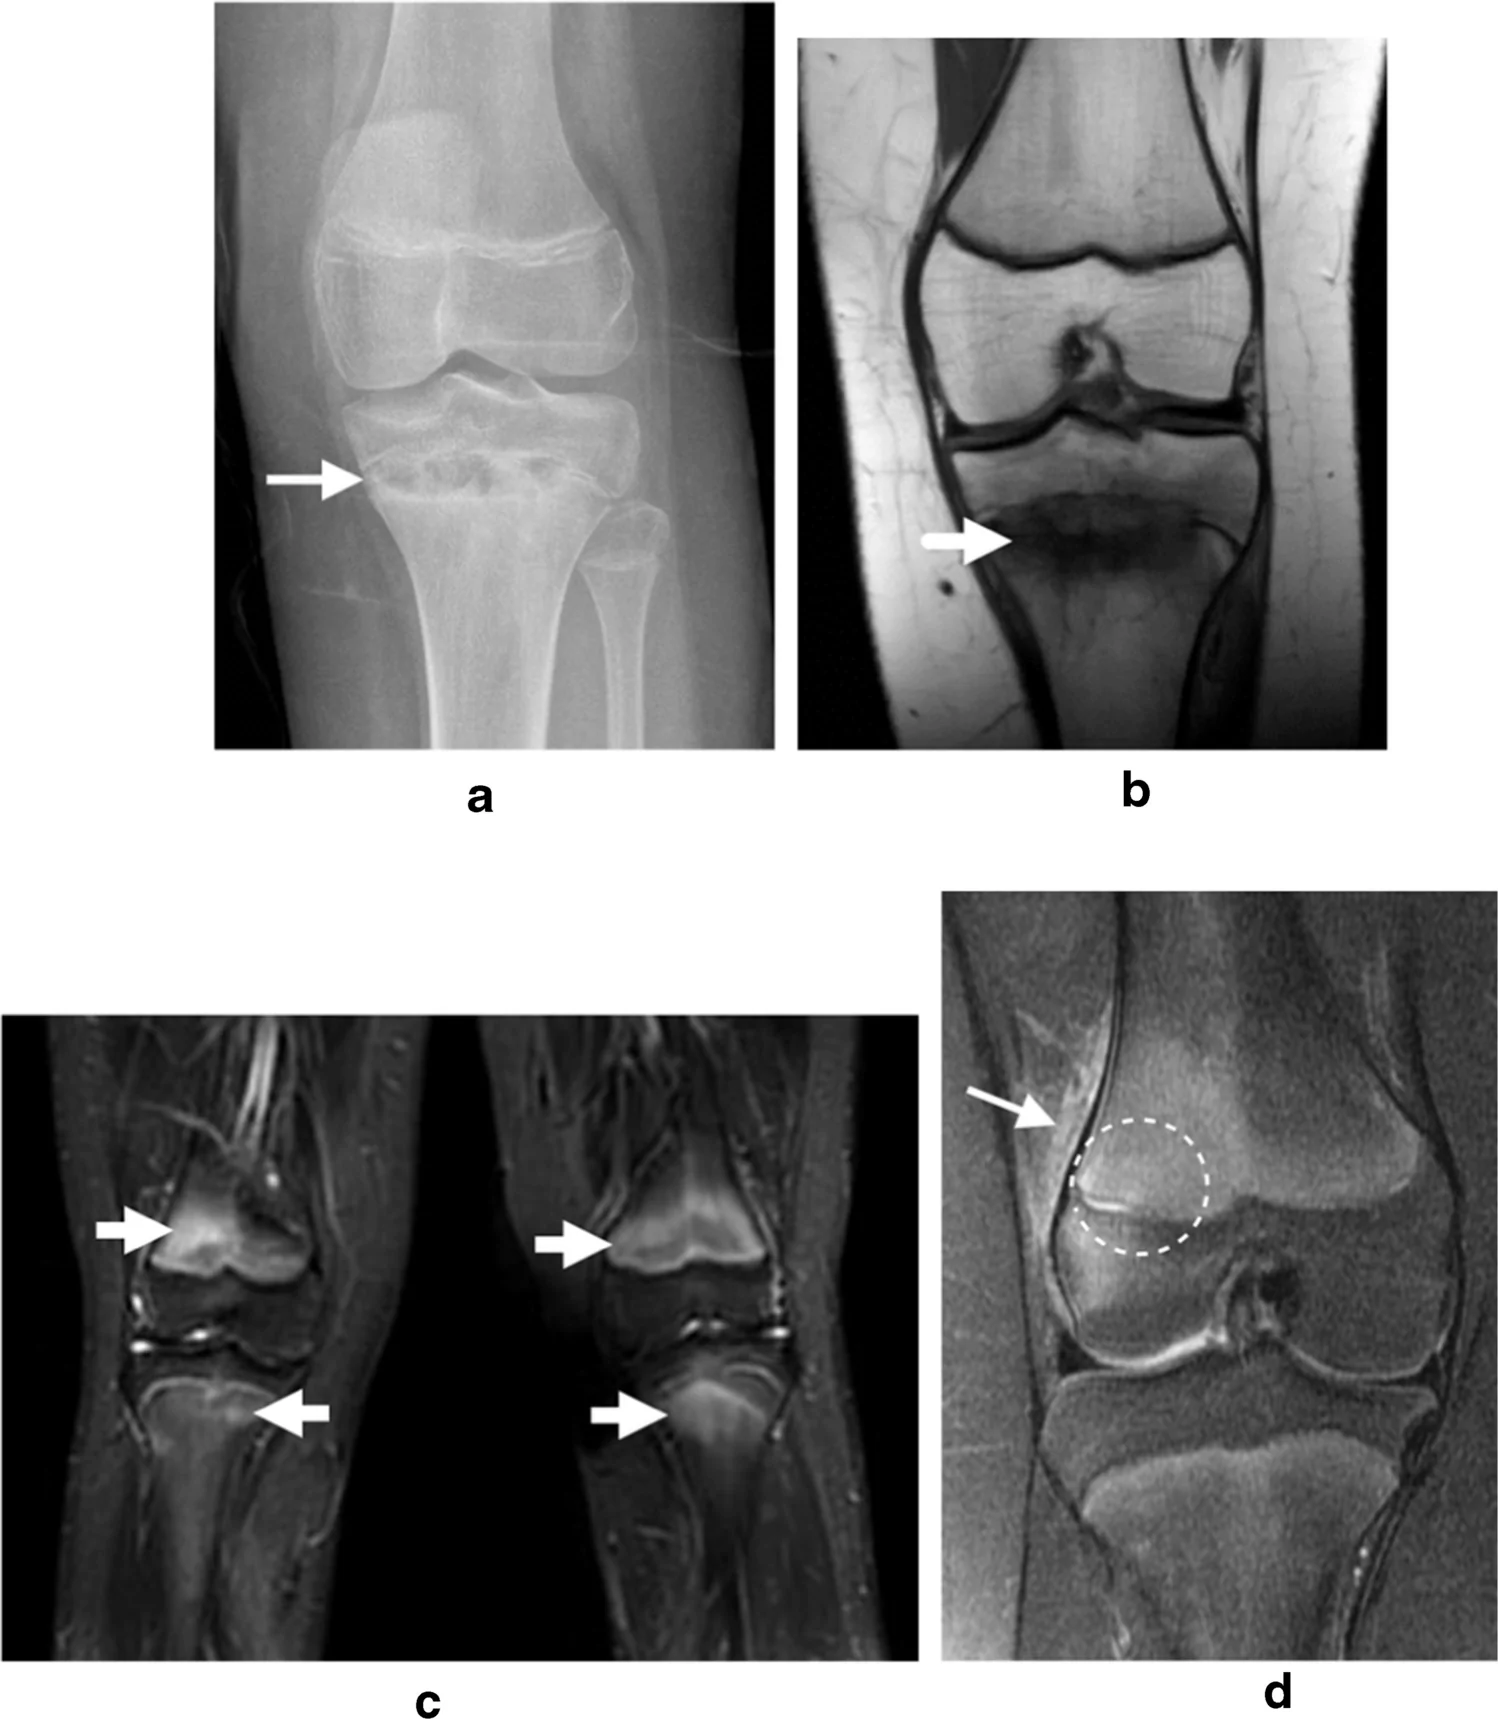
CNO involving the lower extremities. a 10-year-old girl with CNO involving the left leg. Anteroposterior radiograph shows mixed lytic and sclerotic changes in the proximal tibial metaphysis (arrow). b Coronal T1-weighted turbo spin-echo (TSE) MRI demonstrates low marrow signal extending from the proximal tibial metaphysis into the epiphysis with intact physis (arrow). c 3-year-old with extensive workup including biopsy with cytology and culture negative for osteomyelitis, blood cultures, Lyme antibodies, QuantiFERON test for tuberculosis, HLA B27 testing, extensive autoimmune disease evaluation (ANA, RF, Cyclic citrulline peptide IGG among other test) and Vitamin D levels. Coronal T2 STIR MR image of both knees shows areas of hyperintensity involving both distal femoral, and proximal tibial metaphysis (arrows), suggesting CNO. d 11-year-old boy with acute, uncomplicated osteomyelitis of the lateral distal metaphysis and epiphysis of the right femur. Coronal T2 fat-suppressed MR image demonstrates mild physeal widening with hyperintense edema in the lateral distal femur (dashed circle), overlying periostitis, and adjacent soft tissue edema (arrow). Trace joint fluid is present without significant inflammatory changes to suggest septic arthritis
